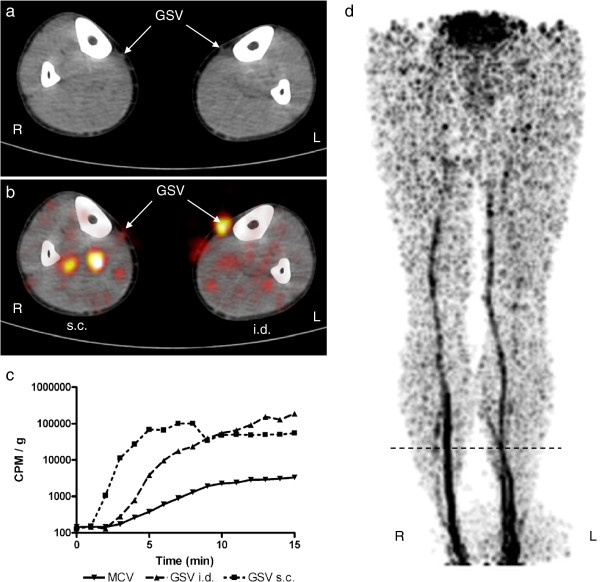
Results for subject 3. For figure details, see legend of Figure 1. A 45-year-old male. On the right lower extremity (s.c. depot), ^18^F-FDG activity is visualized in deep veins, while on the left (i.d. depot), in the great saphenous vein.
